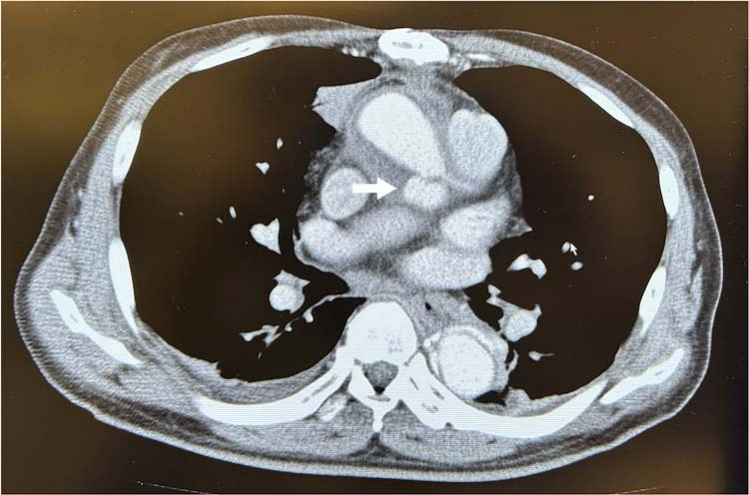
Computed tomography scans revealed a pseudoaneurysm from the left coronary artery. The arrow indicates the pseudoaneurysm.
